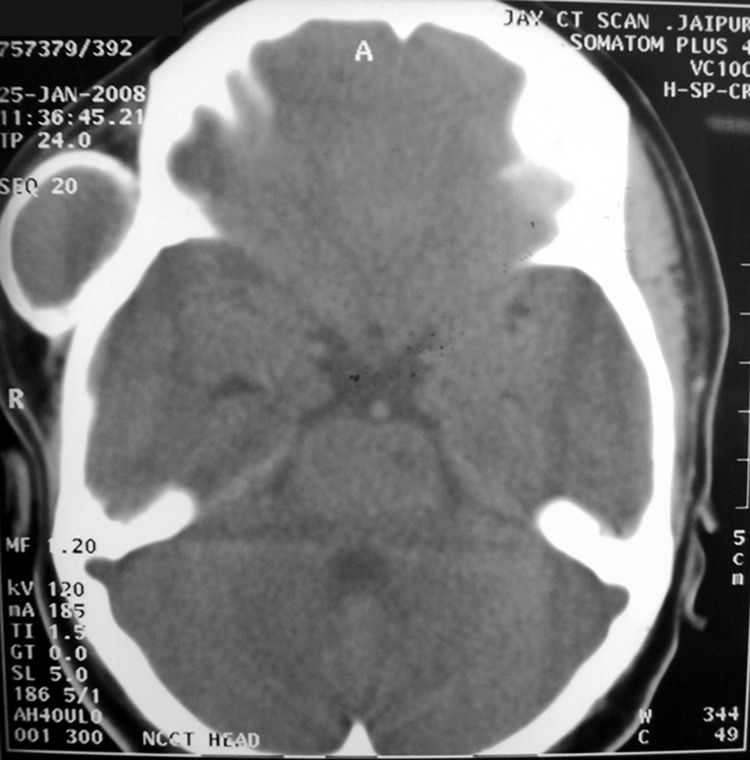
CT scan of secondary lesion in right temporal
